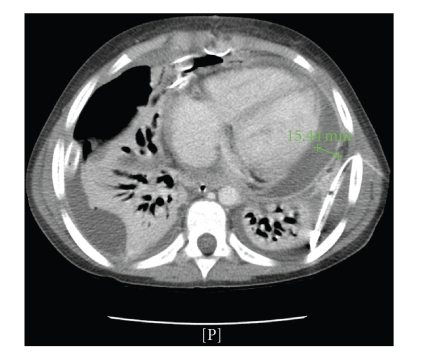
CT scan after creation of a pleuropericardial window on the right side.
